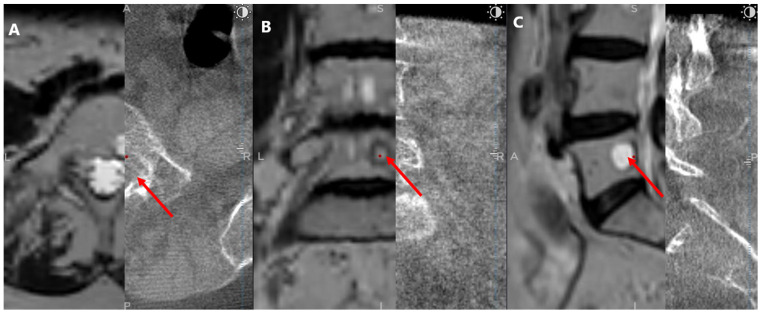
Postbiopsy O-arm and MRI fused image: (A) Axial view; (B) Coronal view; (C) Sagittal view. The left half of each view displays the MRI scan. The right half displays the O-arm scan. Red arrows indicate the biopsy sample location.
